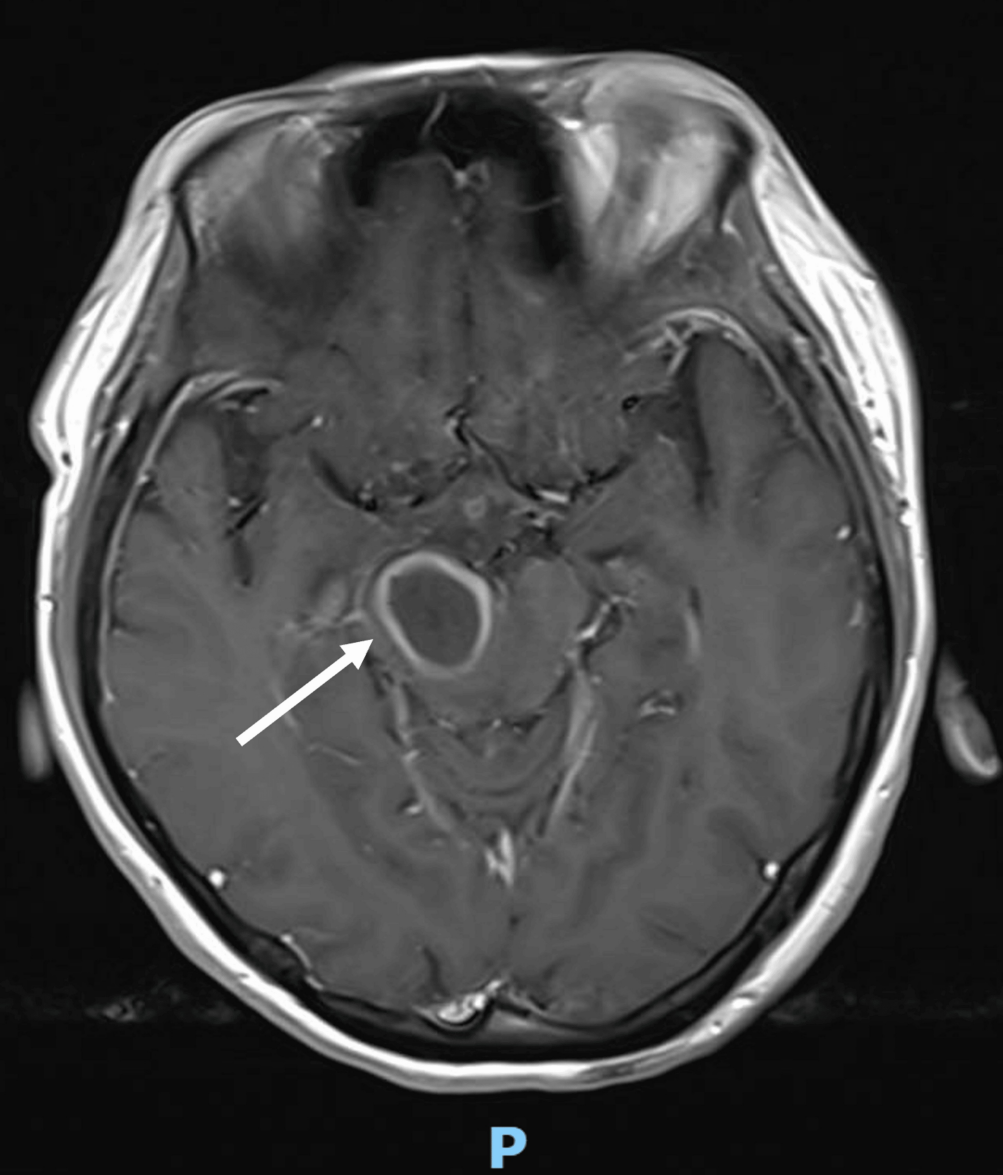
MRI brain demonstrating right midbrain/cerebral peduncle abscess (arrow)
